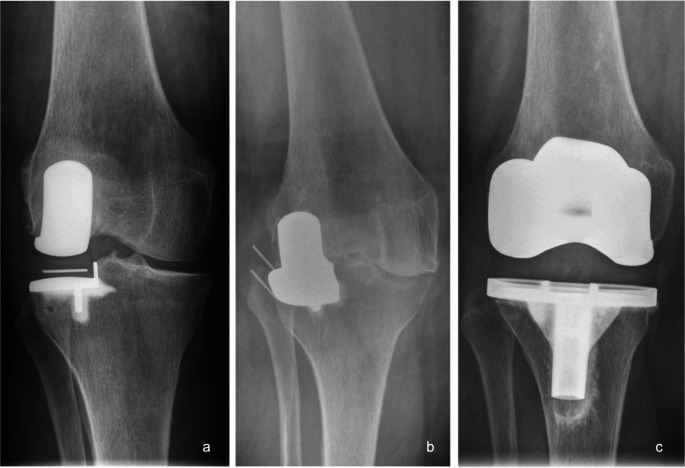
Case history of a 74-year-old female patient who experienced an atraumatic bearing dislocation while standing up from a chair, 16 months after receiving a lateral  UKR. Revision surgery was performed, during which the bearing was exchanged from size 7 to size 8. Thirteen months later, a second bearing dislocation occurred (b), necessitating conversion to a TKR (c), a: AP x-ray shortly after lateral UKR, b: x-ray after the second dislocation, showing lateral displacement with associated movement limitation of the knee, c: AP x-ray after revision surgery to TKR, AP: antero-posterior, TKR: total knee replacement, UKR: unicompartmental knee replacement
